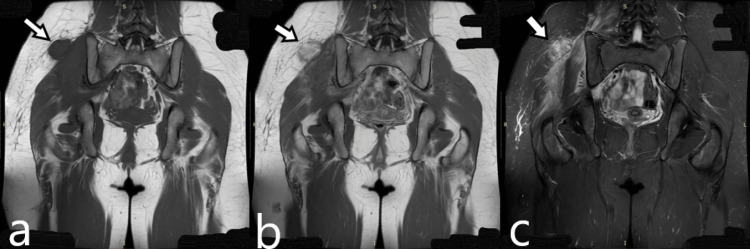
Coronal MRI images (a) Coronal T1W MRI shows a mass in the subcutaneous tissue with signal intensity similar to that of skeletal muscle, without clear boundaries and in contact with the gluteus muscle, measuring 3.8 × 2.3 cm. (b) Coronal contrast-enhanced T1W MRI shows an intensely heterogeneous enhancement of the mass. (c) Coronal FSE STIR MRI shows marked edema of the gluteus medius and minimus muscles and less intense edema in the paravertebral muscles that had the normal striation. FSE, fast spin echo
